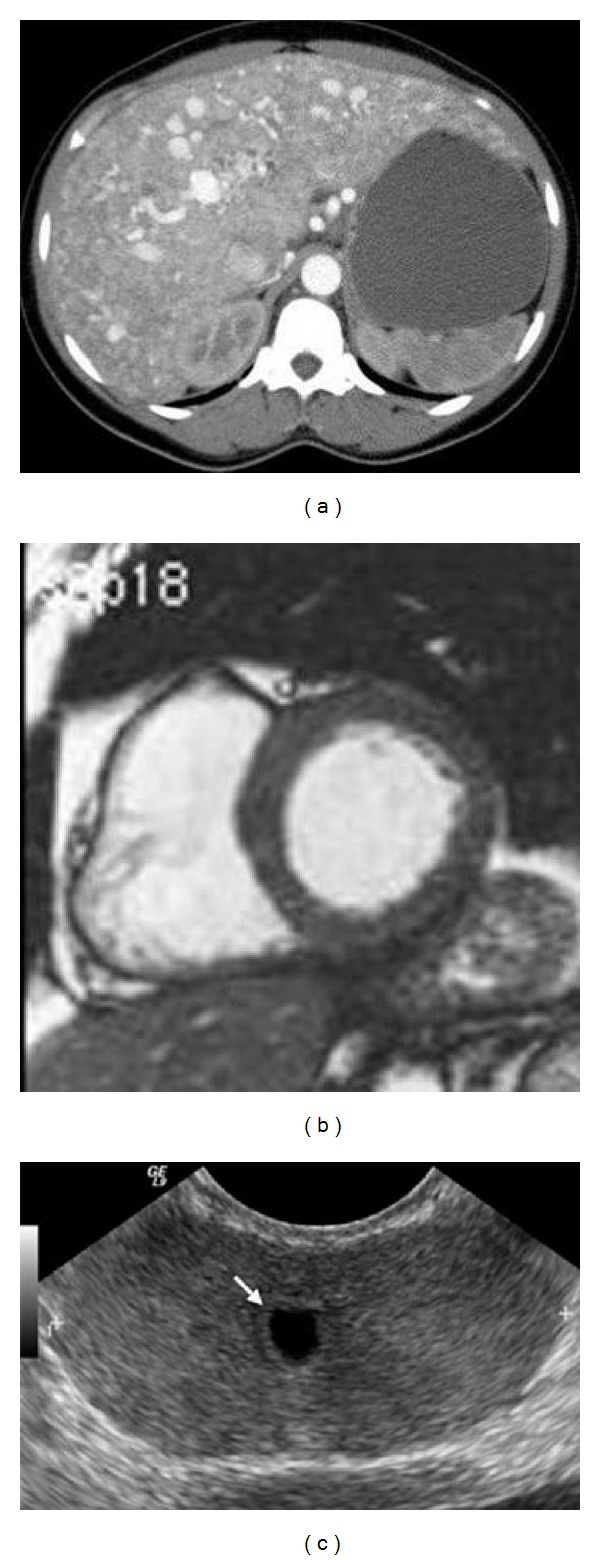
Clinical medical images in treatment planning: (a) contrast-enhanced axial CT scans through liver, (b) left ventricle in cardiac from MRI images and (c) transrectal ultrasound image of the prostate.
